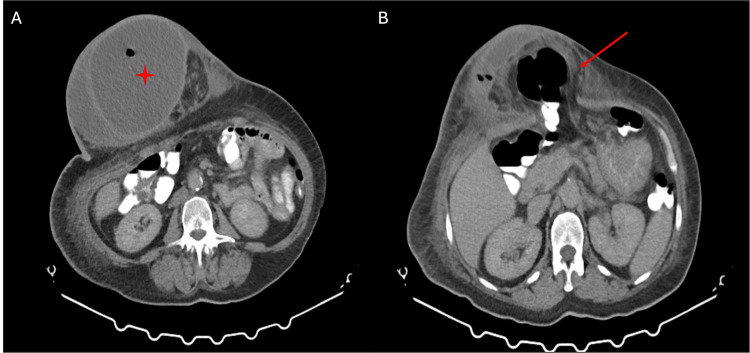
Contrast-enhanced computed tomography scan of the abdomen. (A) Axial view showing the extensive, multiloculated fluid collection and gas bubbles within the subcutaneous tissue of the epigastrium, consistent with a massive abscess (red star). (B) Axial view demonstrating a large fascial defect in the linea alba with herniation of the transverse colon (red arrow).
